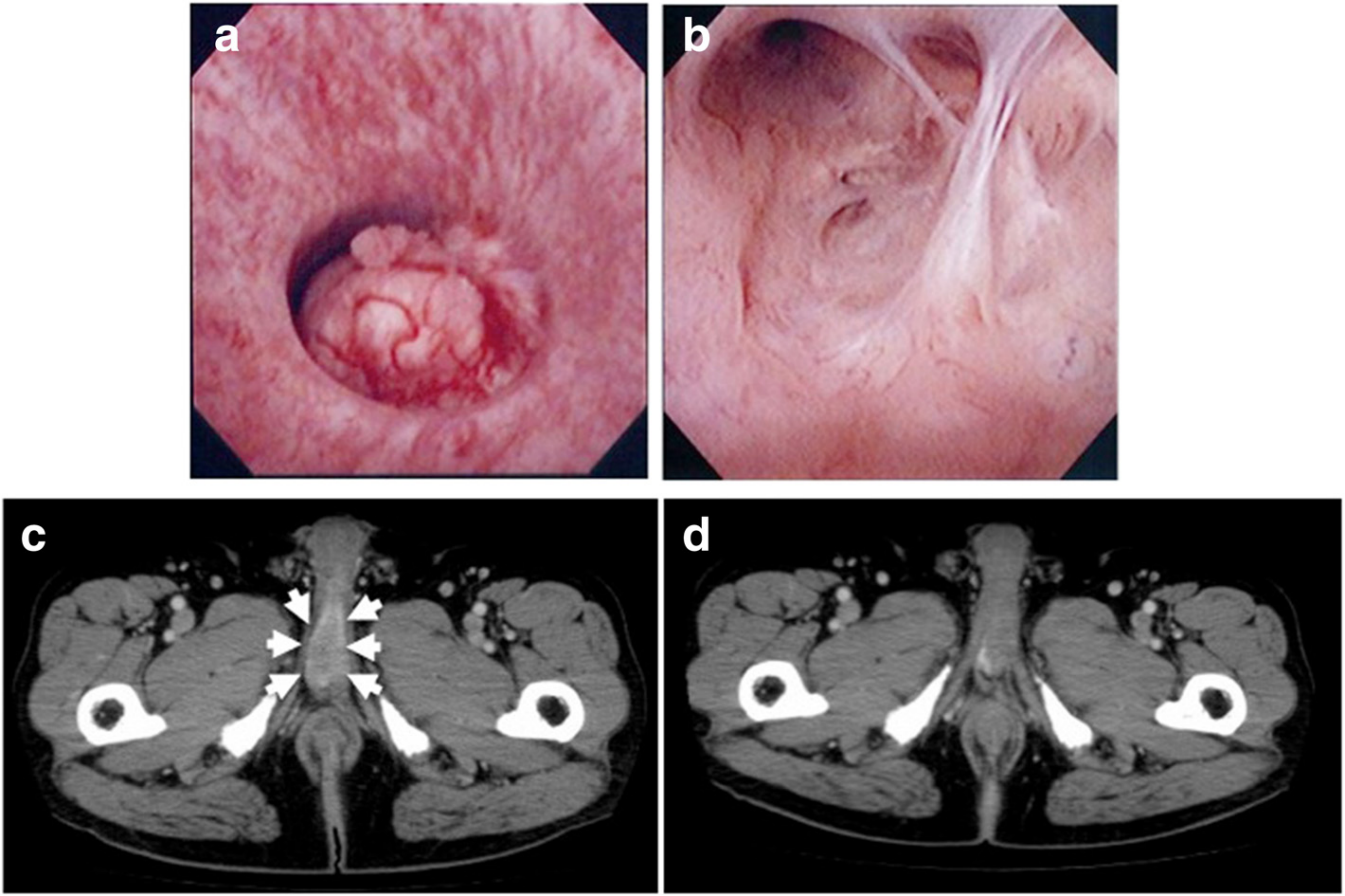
Urethral tumor detected four years after sigmoidectomy. (a) Cystoscopy demonstrated papillary tumor of approximate 7 mm in the urethral wall of the distal-potion from the urethral sphincter. (b) Cystoscopy demonstrated scar of the transurethral resection without recurrence of the tumor. (c) CT-scan imaging showed the small mass of bulbous portion of urethra (white arrow). (d) CT-scan imaging showed the total disappearance of urethral metastasis.
